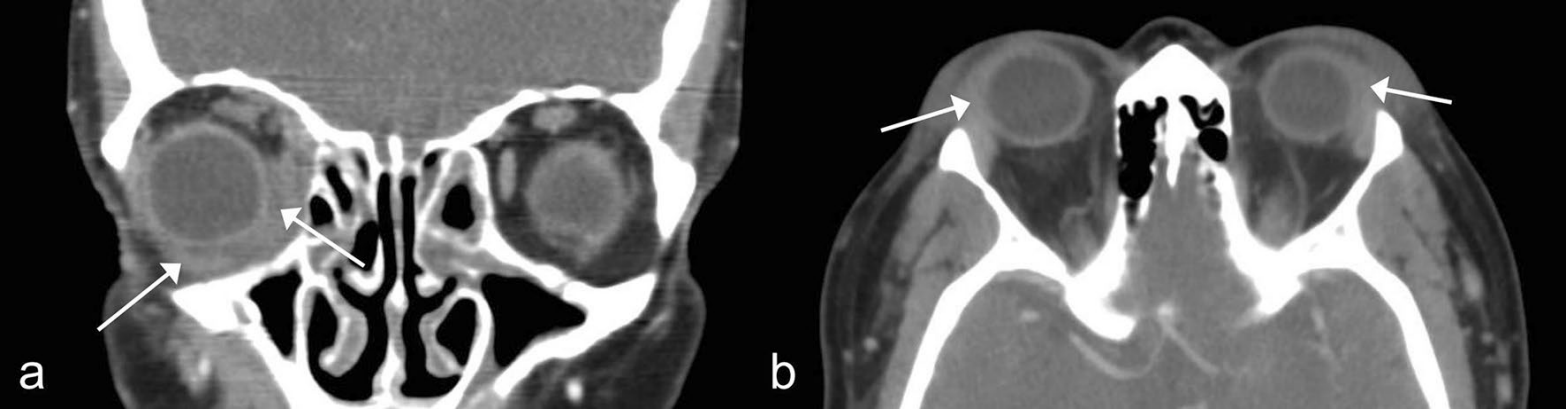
Representative images of non-specific orbital inflammation (NSOI). (A) Coronal, contrast-enhanced computed tomography (CT) image showed inferonasal diffuse infiltrative mass involving inferior rectus and medial rectus muscles (white arrows). The clinical and radiological diagnosis was NSOI. (B) Fusiform mild enlargement of both lacrimal glands shows isodensity and minimal enhancement on contrast-enhanced CT (white arrows). Preseptal soft tissue swelling is also present. The clinical diagnosis was lacrimal NSOI, compatible with the radiologic diagnosis.
